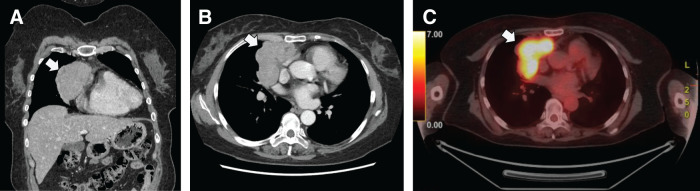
(A, B) Chest CT findings. An 85-mm mass in the anterior mediastinum with a lobulated contour and heterogeneous internal enhancement. The tumor was adjacent to the pericardium and the right upper and right middle lung lobes. (C) 18F-fluorodoeoxyglucose PET/CT showed increased 18F-fluorodoeoxyglucose uptake (maximum standardized uptake value [SUVmax], 12.8) at the tumor site.
